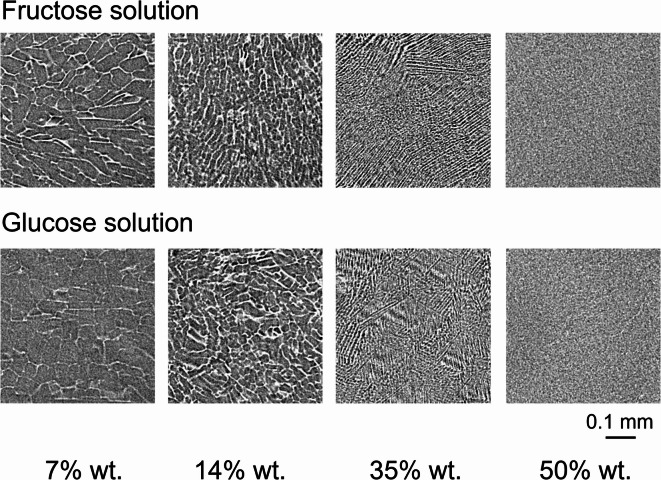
Cross-sectional images of slowly frozen fructose (top row) and glucose solution (bottom row) at different concentrations. The higher the concentration, the finer the stripe spacing, and at 50% the micro- X-ray CT used was unable to resolve the stripes, resulting in a coarse sand-like pattern being observed.
